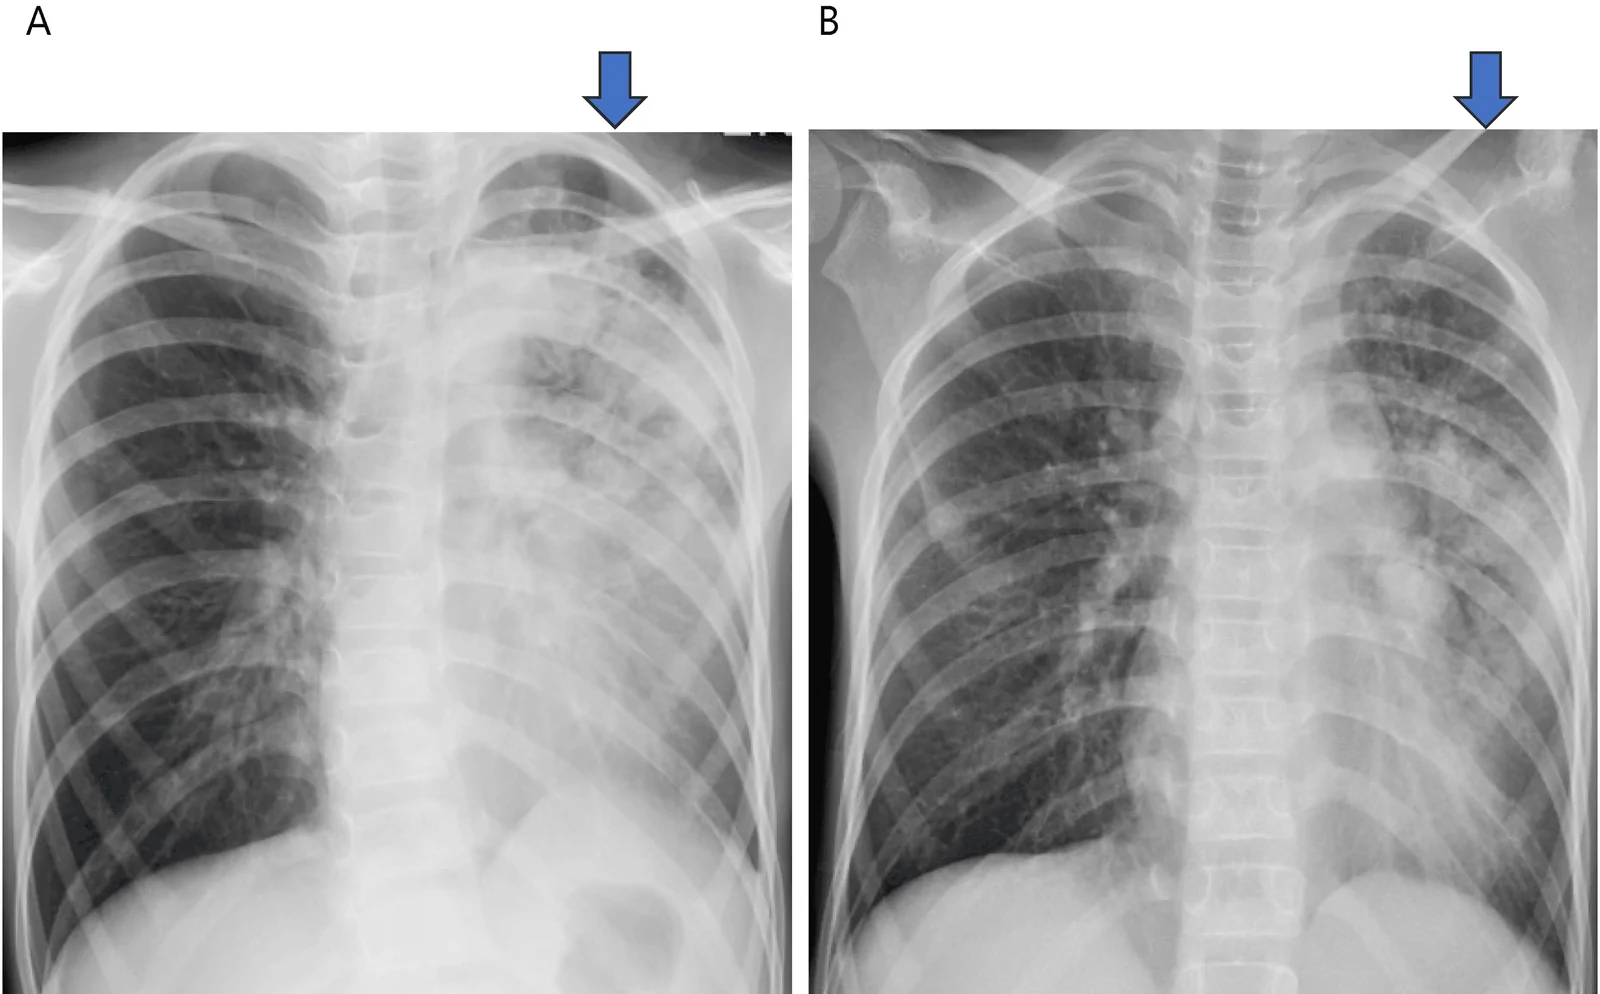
Chest radiography (A) Extensive ground-glass opacities are observed in the left lung field on admission. (B) Ground-glass opacity in the left lung field has improved significantly.
